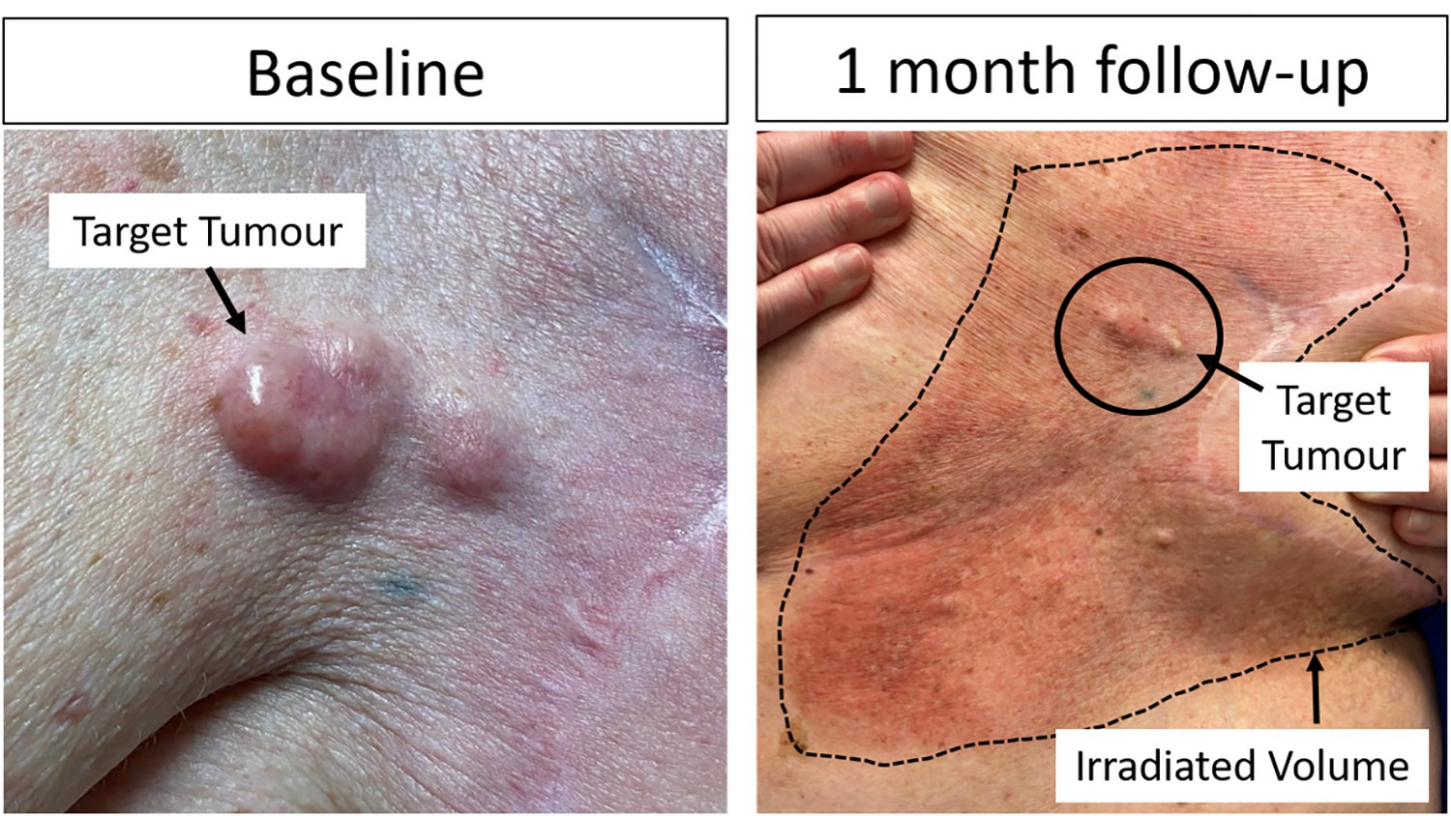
Example of radiation dermatitis following focussed ultrasound-stimulated microbubbles radiation enhancement treatment. A patient with cutaneous skin nodules (identified as patient #9 in Tables 1 and 2) received radiation therapy comprising 20 Gy in 5 fractions with electrons to the area delineated by a dotted line. The nodule, identified at baseline image and demarcated by a solid black line at the one-month follow-up, was the target for radioenhancement treatment with MRgFUS-MB. The most severe observed toxicity was grade 1 dermatitis, and the region treated with combined treatment (MRgFUS-MB + radiation) displayed a similar intensity of cutaneous toxicity compared to the areas treated with radiotherapy alone, suggesting that radiation dermatitis would likely have occurred to a comparable extent in the absence of radioenhancement therapy.
